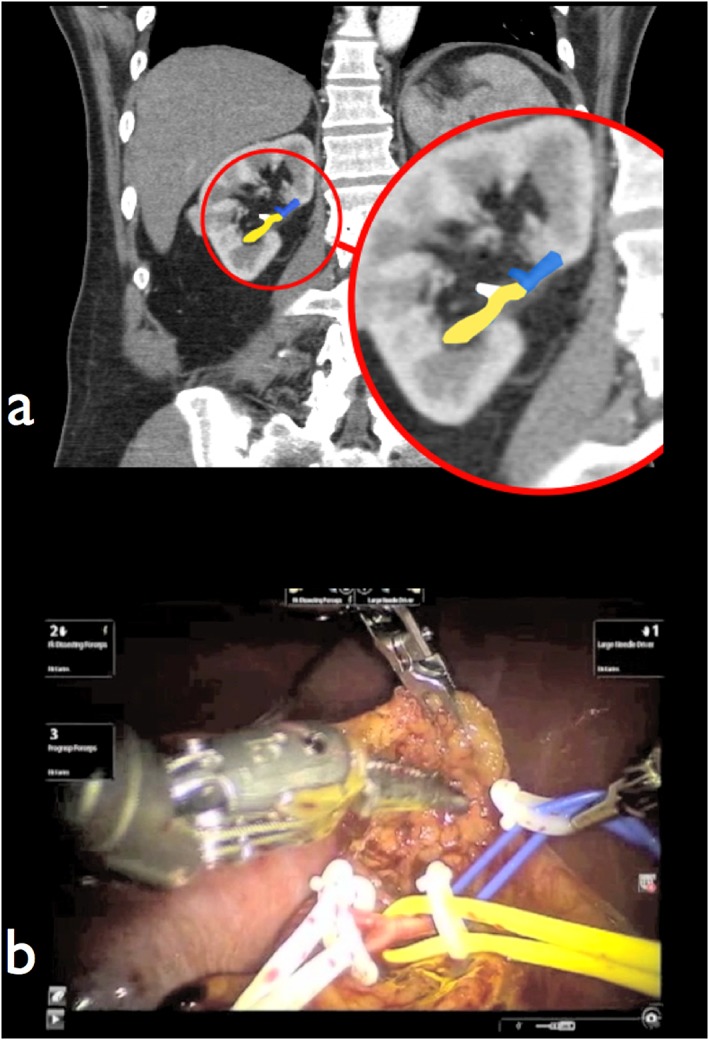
(a) Coronal CT scan of right kidney demonstrating colour‐coded primary or anterior (blue), secondary (yellow) and tertiary (white) divisions of the right renal artery. (b) Equivalent intraoperative image with the same colour coded plastic slings round the corresponding vessels
